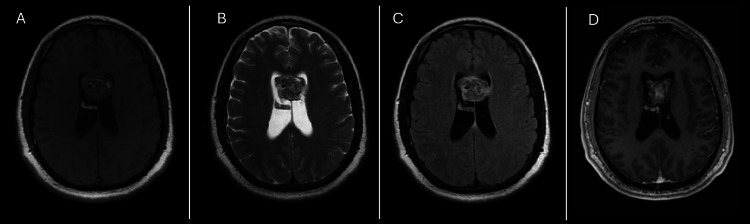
MRI Characteristics of Midline Intraventricular Lesion Involving the Septum Pellucidum and Corpus Callosum Brain MRI showing a lesion of 2.5 × 3.0 × 2.1 cm located in the midline anterior body and frontal horns of the lateral ventricles straddling the septum pellucidum and extending into the splenium of the corpus callosum with heterogeneous hyperintense signal on the T1 imaging (A), mixed signal intensity on T2 sequence as in FLAIR (B, C), and bubbly heterogenous enhancing lesion in the T1 with contrast sequence (D).
